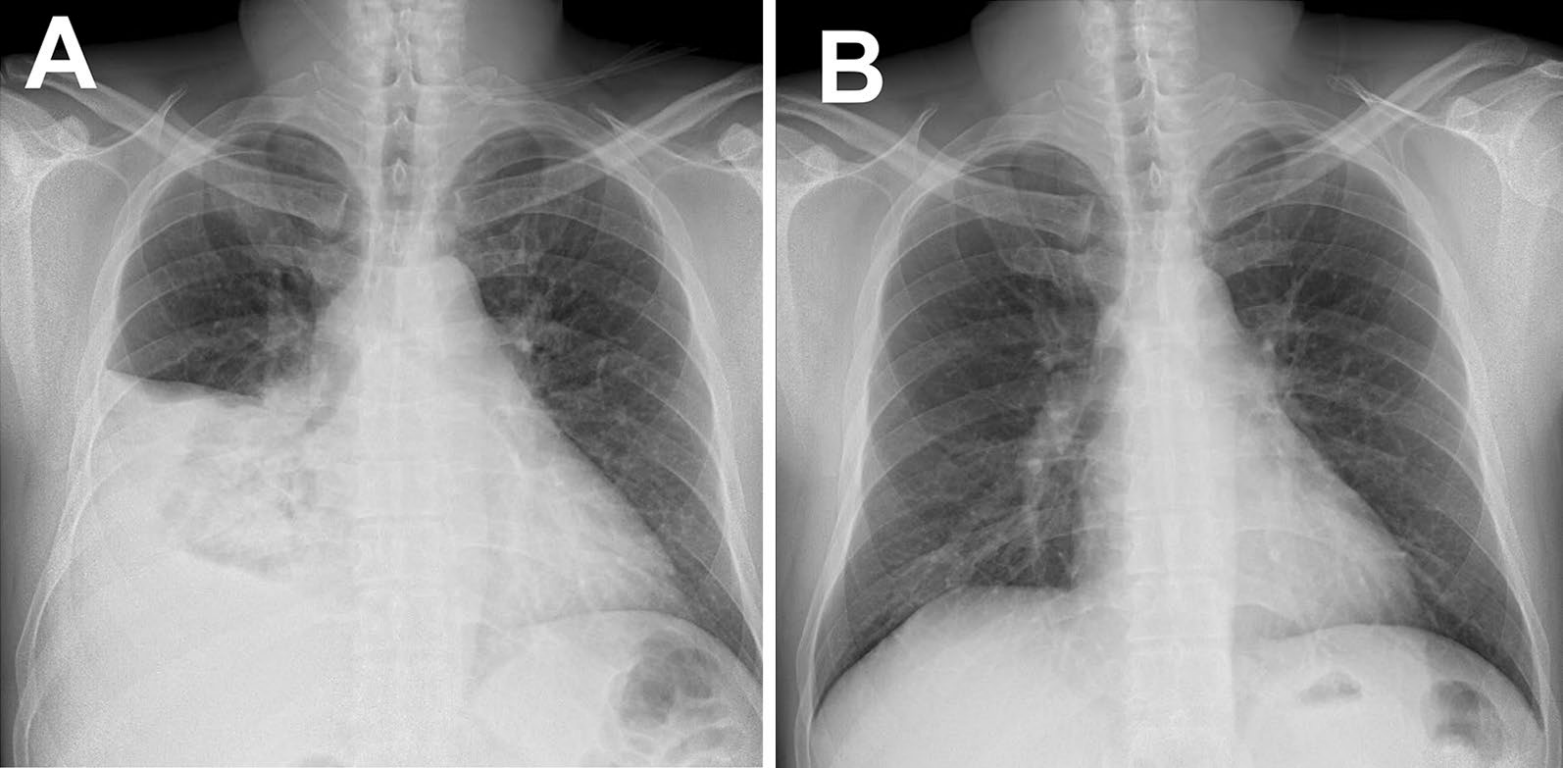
Follow-up chest X-rays. A chest X-ray on hospital day 2 showed rapid progression of the consolidation and pleural effusion (A). After administration of ribavirin, the lesions were completely resolved on hospital day 13 (B)
